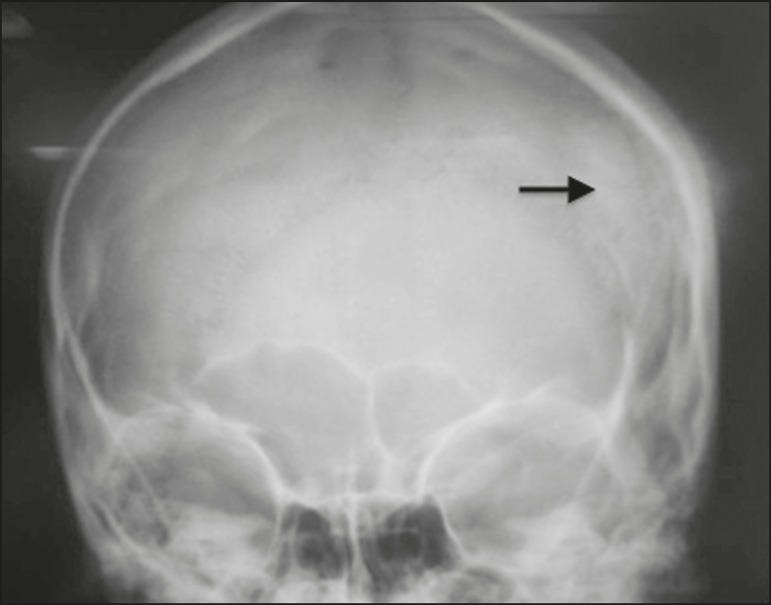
Anteroposterior X-ray of the skull, showing a heterogeneous
